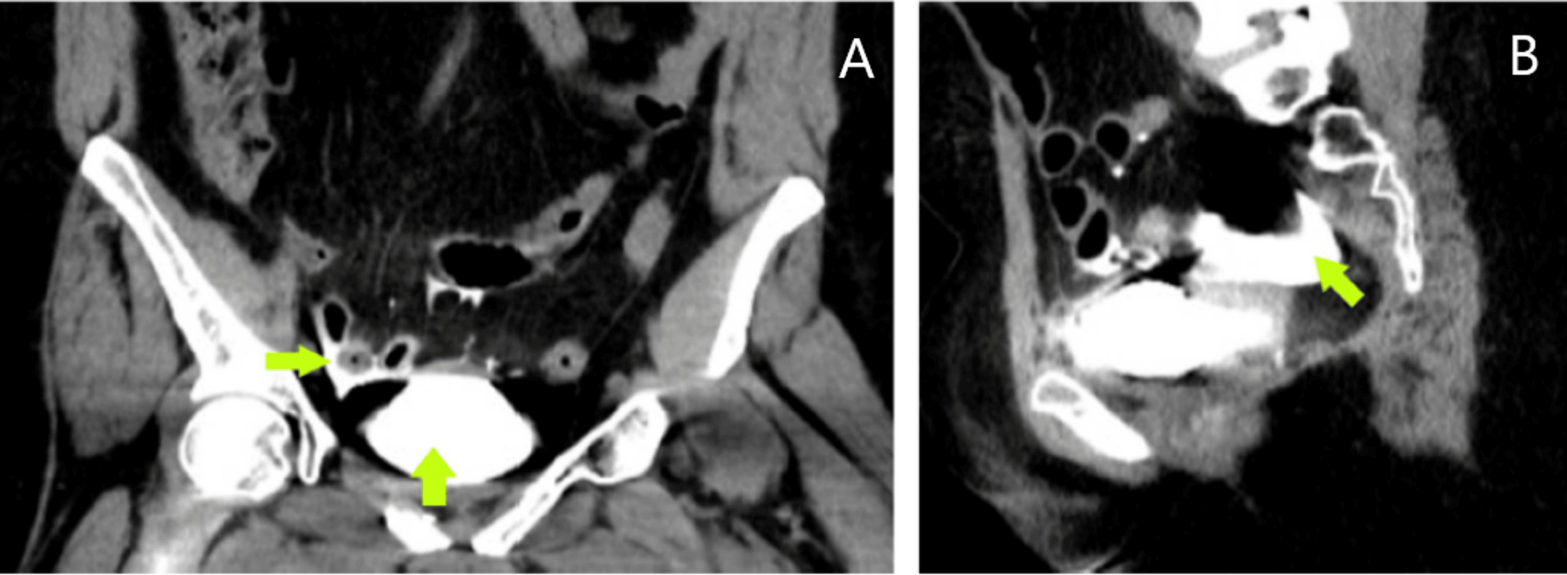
Follow-up CT scan after the administration of contrast fluid through the urinary catheter Abdominal CT showing intraperitoneal extravasation of contrast fluid (marked by the green arrows). Sub-image A shows the coronal plane, while sub-image B shows the sagittal plane.
